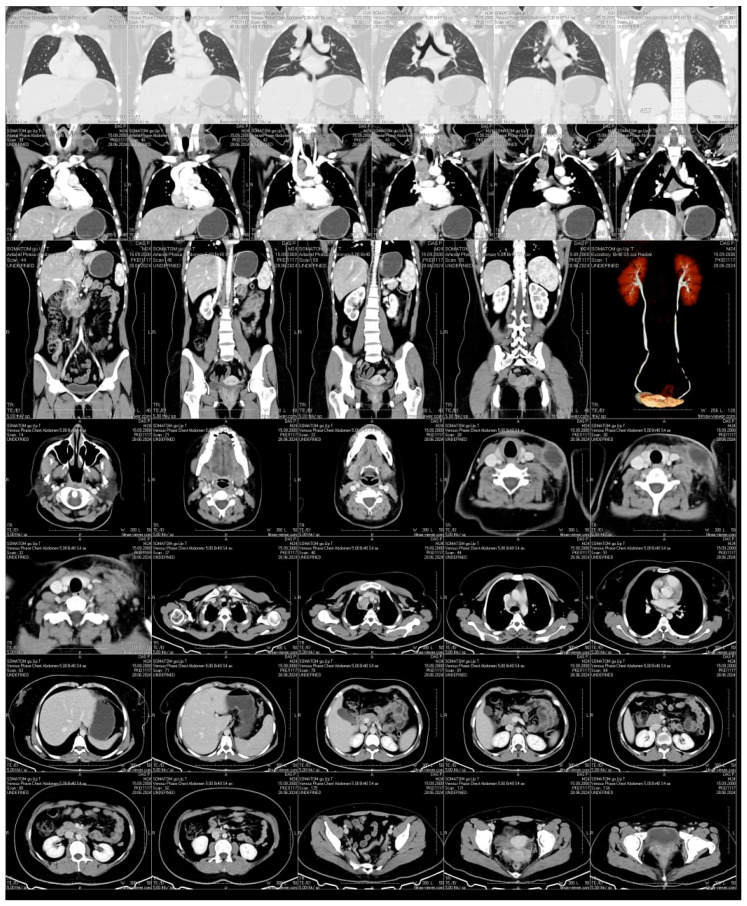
Contrast-Enhanced CT Scan. An encapsulated fluid collection with well-defined margins is visualized in the left supraclavicular region, consistent with an abscess or a necrotically transformed lymph node. Prominent lymphadenopathy is also observed in the paratracheal and subcarinal regions, with evidence of central necrosis. No signs of pulmonary involvement or abdominal pathology are detected.
